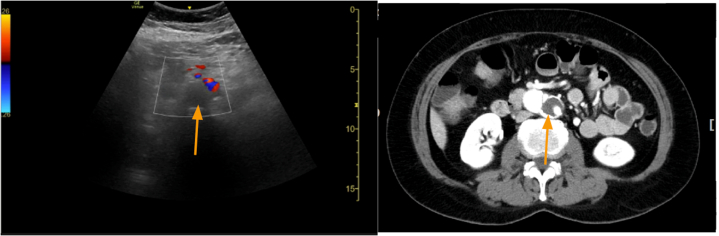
Point-of-care ultrasonography with color Doppler (left) showed a filling defect in the abdominal aorta (arrow), compatible with the findings in computed tomography angiography (right).
